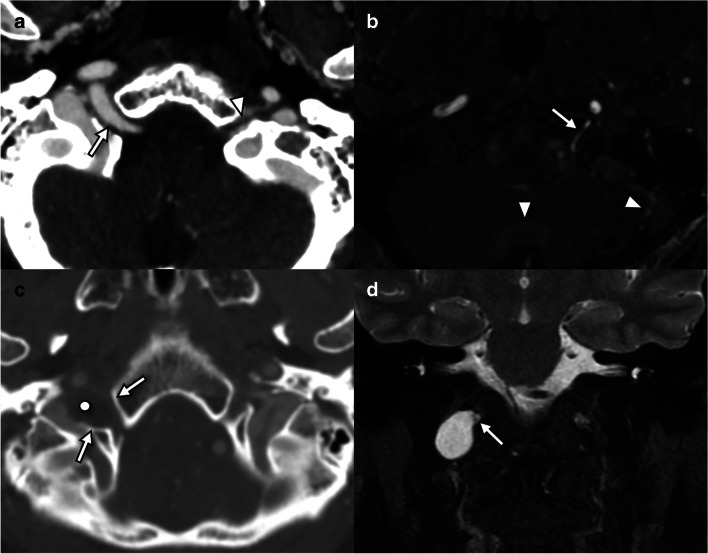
Lesions of the hypoglossal canal which are not commonly associated with denervation. a Axial CT angiography in an adult patient presenting with transient ischemic attack demonstrates an artery coursing through the right hypoglossal canal (arrow) forming an anastomosis between the right internal carotid artery and the basilar trunk (not shown), a persistent primitive hypoglossal artery, and a congenital anomaly. Note the normal left hypoglossal nerve (arrowhead), seen as a filling defect surrounded by venous plexus. This patient did not have hypoglossal palsy. b Axial time-of-flight MR angiogram in a 73-year-old (male) with pulsatile tinnitus demonstrates a high flow vessel traversing the left hypoglossal canal (arrow) and with arterialized flow in the dural venous sinuses (arrowheads). DSA (not shown) revealed multiple dural arteriovenous fistulas. This patient did not have hypoglossal palsy. c Axial CT angiography in a 59-year-old (female) presenting with an episode of dizziness reveals smooth remodeling of the right hypoglossal canal (arrows) due to a hypoattenuating cystic lesion (dot). d Coronal T2W MRI in the same patient as c demonstrates a T2 hyperintense circumscribed lesion protruding through the right hypoglossal canal (arrow), consistent with perineural sleeve cyst. It was isointense to CSF on all sequences and did not demonstrate any enhancement or reduced diffusion (not shown). This patient did not have hypoglossal palsy
